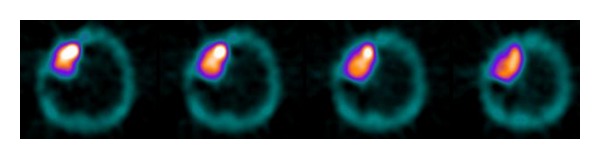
Transverse slices of a ^99m^Tc-tetrofosmin SPECT brain study in a patient with meningioma showing increased tracer uptake by the tumor.
